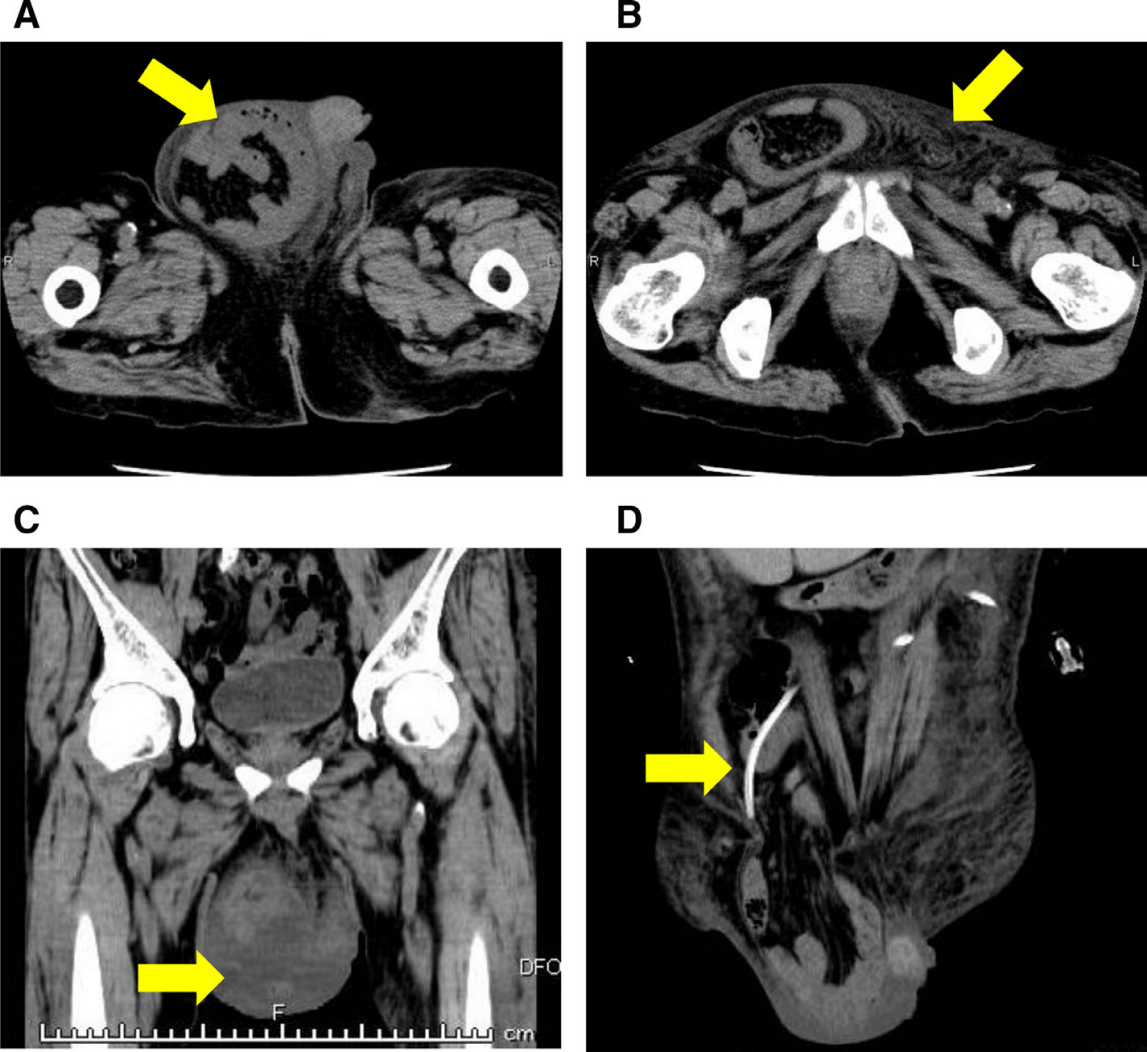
CT findings. (A) Small bowel within the inguinal hernia sac (yellow arrow). (B) Subcutaneous edema in the groin area (yellow arrow). (C) Hydrocele within the scrotum (yellow arrow). (D) The PD catheter tip remained in an appropriate intraperitoneal position without migration into the hernia sac (yellow arrow). PD, peritoneal dialysis
